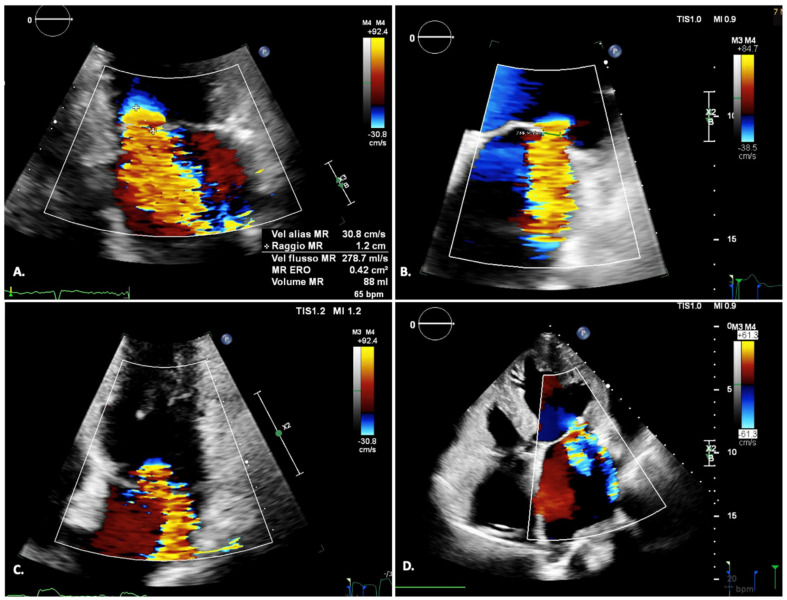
Echocardiographic severity parameters for mitral regurgitation; (A) Quantitative parameter: EROA; (B) Semi-quantitative parameter: Vena contracta; (C,D) Qualitative parameters: Color flow, Coanda effect.
